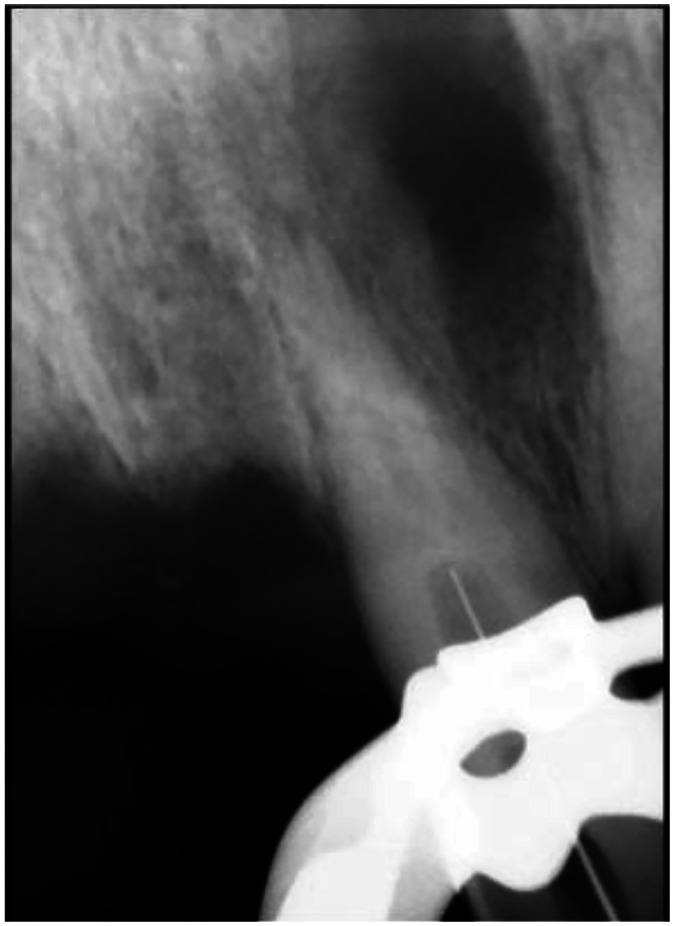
Periapical radiography during treatment.
